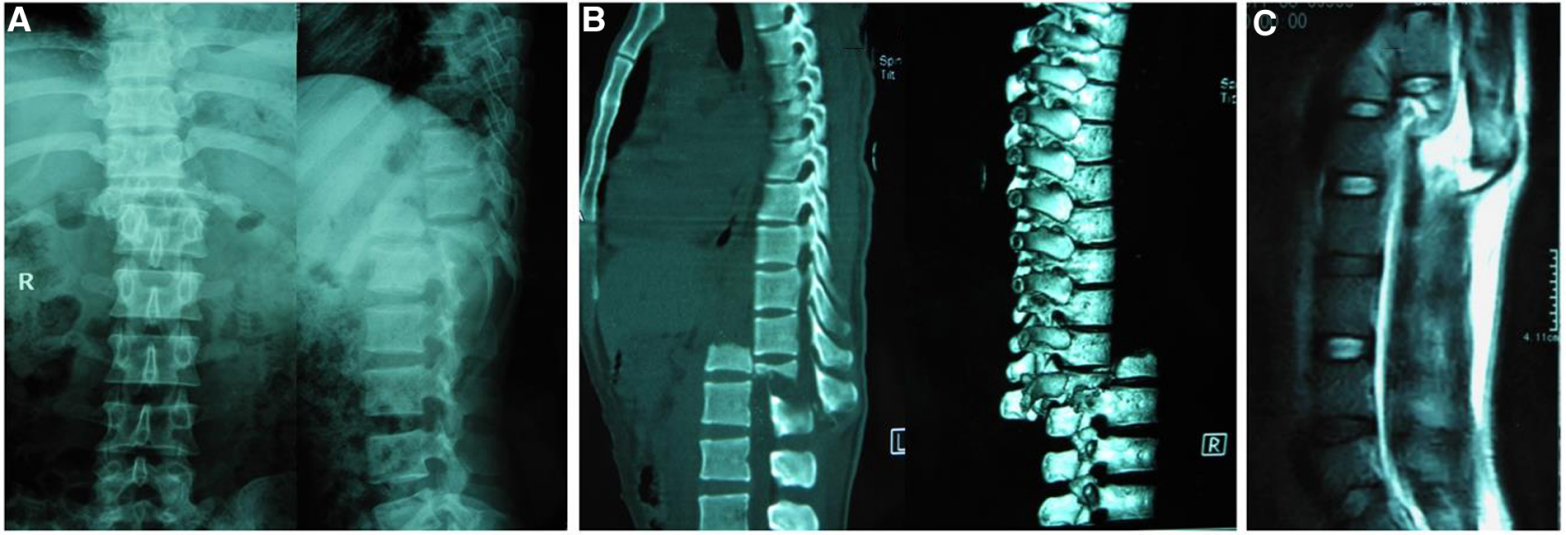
Preoperative x-ray (A) and reconstruction 3D CT (B) depicted complete posterior spondylopotosis of the upper 1/3 T12 overriding to the lower part of T12. Sagittal T2-weighted MRI (C) depicted a completely distorted thecal sac and spinal cord.
